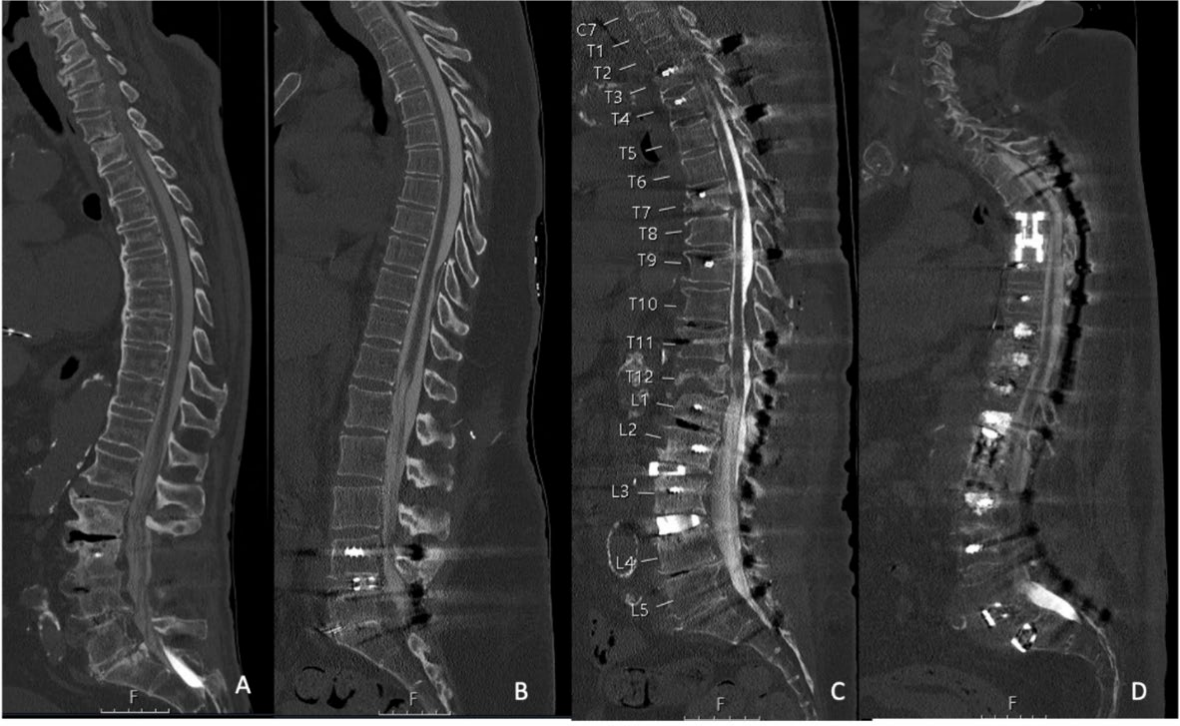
Examples for computed tomography (CT) myelography scans (after intradural contrast agent administration) in different patients performed with a standard-dose (SD) protocol for scanning, which were rated with perfect (A; L = 750, W = 2500, 140 kV, 319 mA, bone kernel; 2 mm, iDose4, CTDI_vol_ 13.4 mGy), good (B; L = 750, W = 2500, 120 kV, 494 mA, bone kernel; 2 mm, iDose4, CTDI_vol_ 19.7 mGy), medium (C; L = 750, W = 2500, 120 kV, 347 mA, bone kernel; 2 mm, iDose4, CTDI_vol_ 18.2 mGy), and poor (D; L = 750, W = 2500, 140 kV, 418 mA, bone kernel; 2 mm, iDose4, CTDI_vol_ 38.8 mGy) overall image quality.
